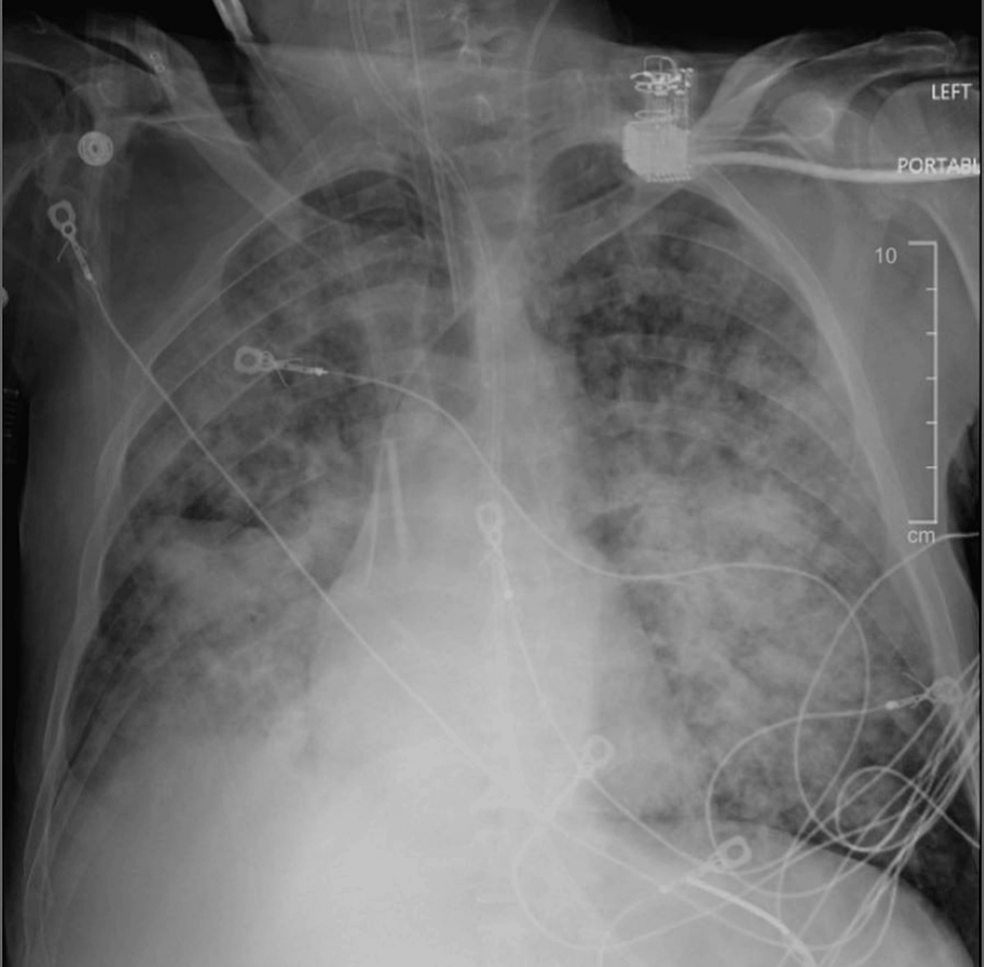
Chest X-ray revealing diffuse pulmonary opacities.
